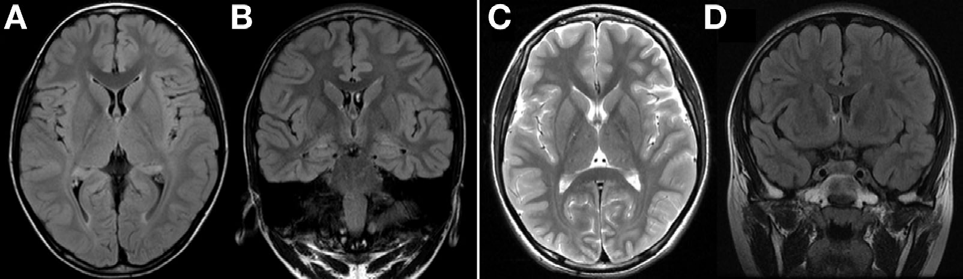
(A,B) Axial and coronal FLAIR MRI at 4 years of age showed normal findings. (C,D) Axial T2-weighted MRI and coronal FLAIR MRI at 6 years of age showed no significant interval change. FLAIR, fluid attenuated inversion recovery; MRI, magnetic resonance imaging.
